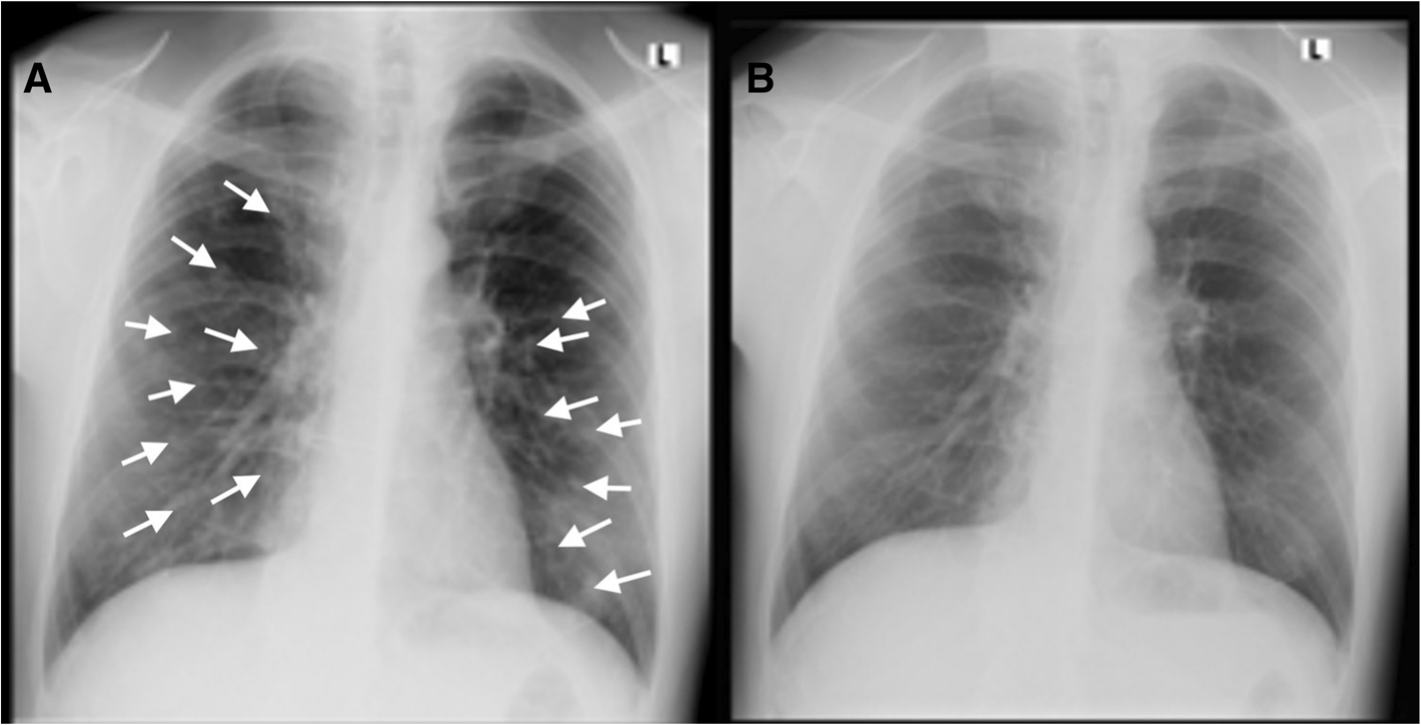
a Chest radiograph on the day of admission revealed diffuse nodular infiltrates. b Radiological resolution of pulmonary infiltrates after 10 days of acyclovir therapy
